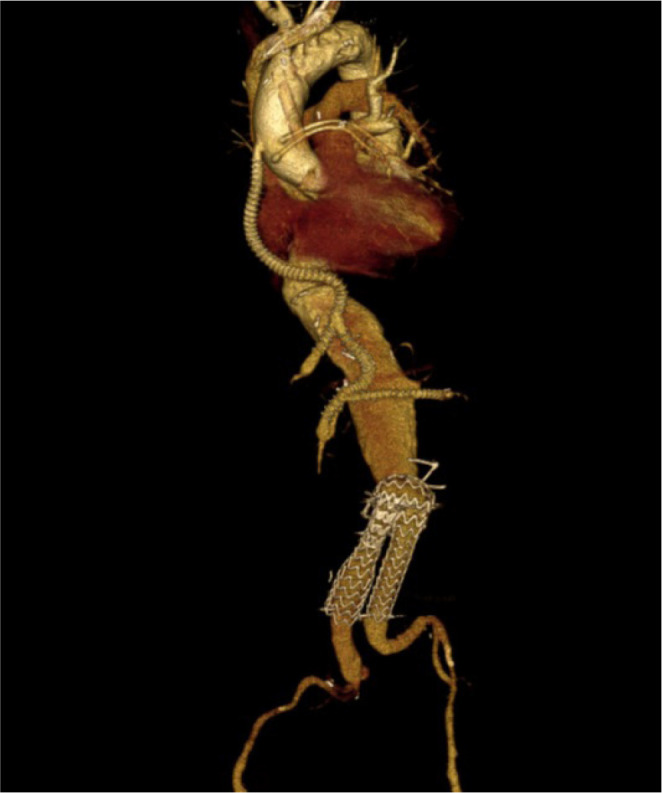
Postoperative computed tomography angiography of the patient.
